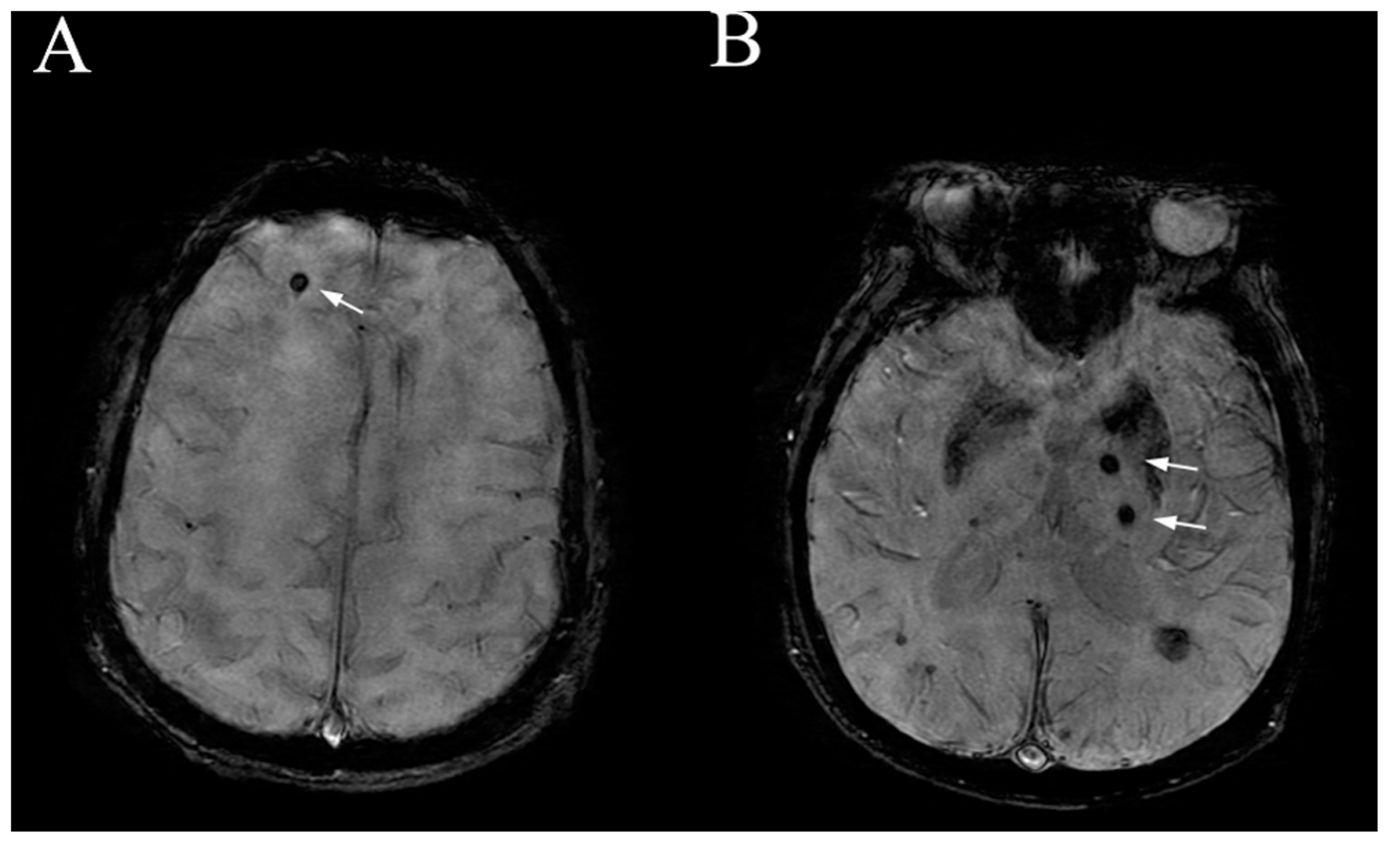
The location of cerebral microbleeds (CMBs) on 3D-enhanced T2*-weighted gradient echo sequence of MRI (arrows). (A) CMBs in frontal lobe. (B) CMBs in thalamus.
